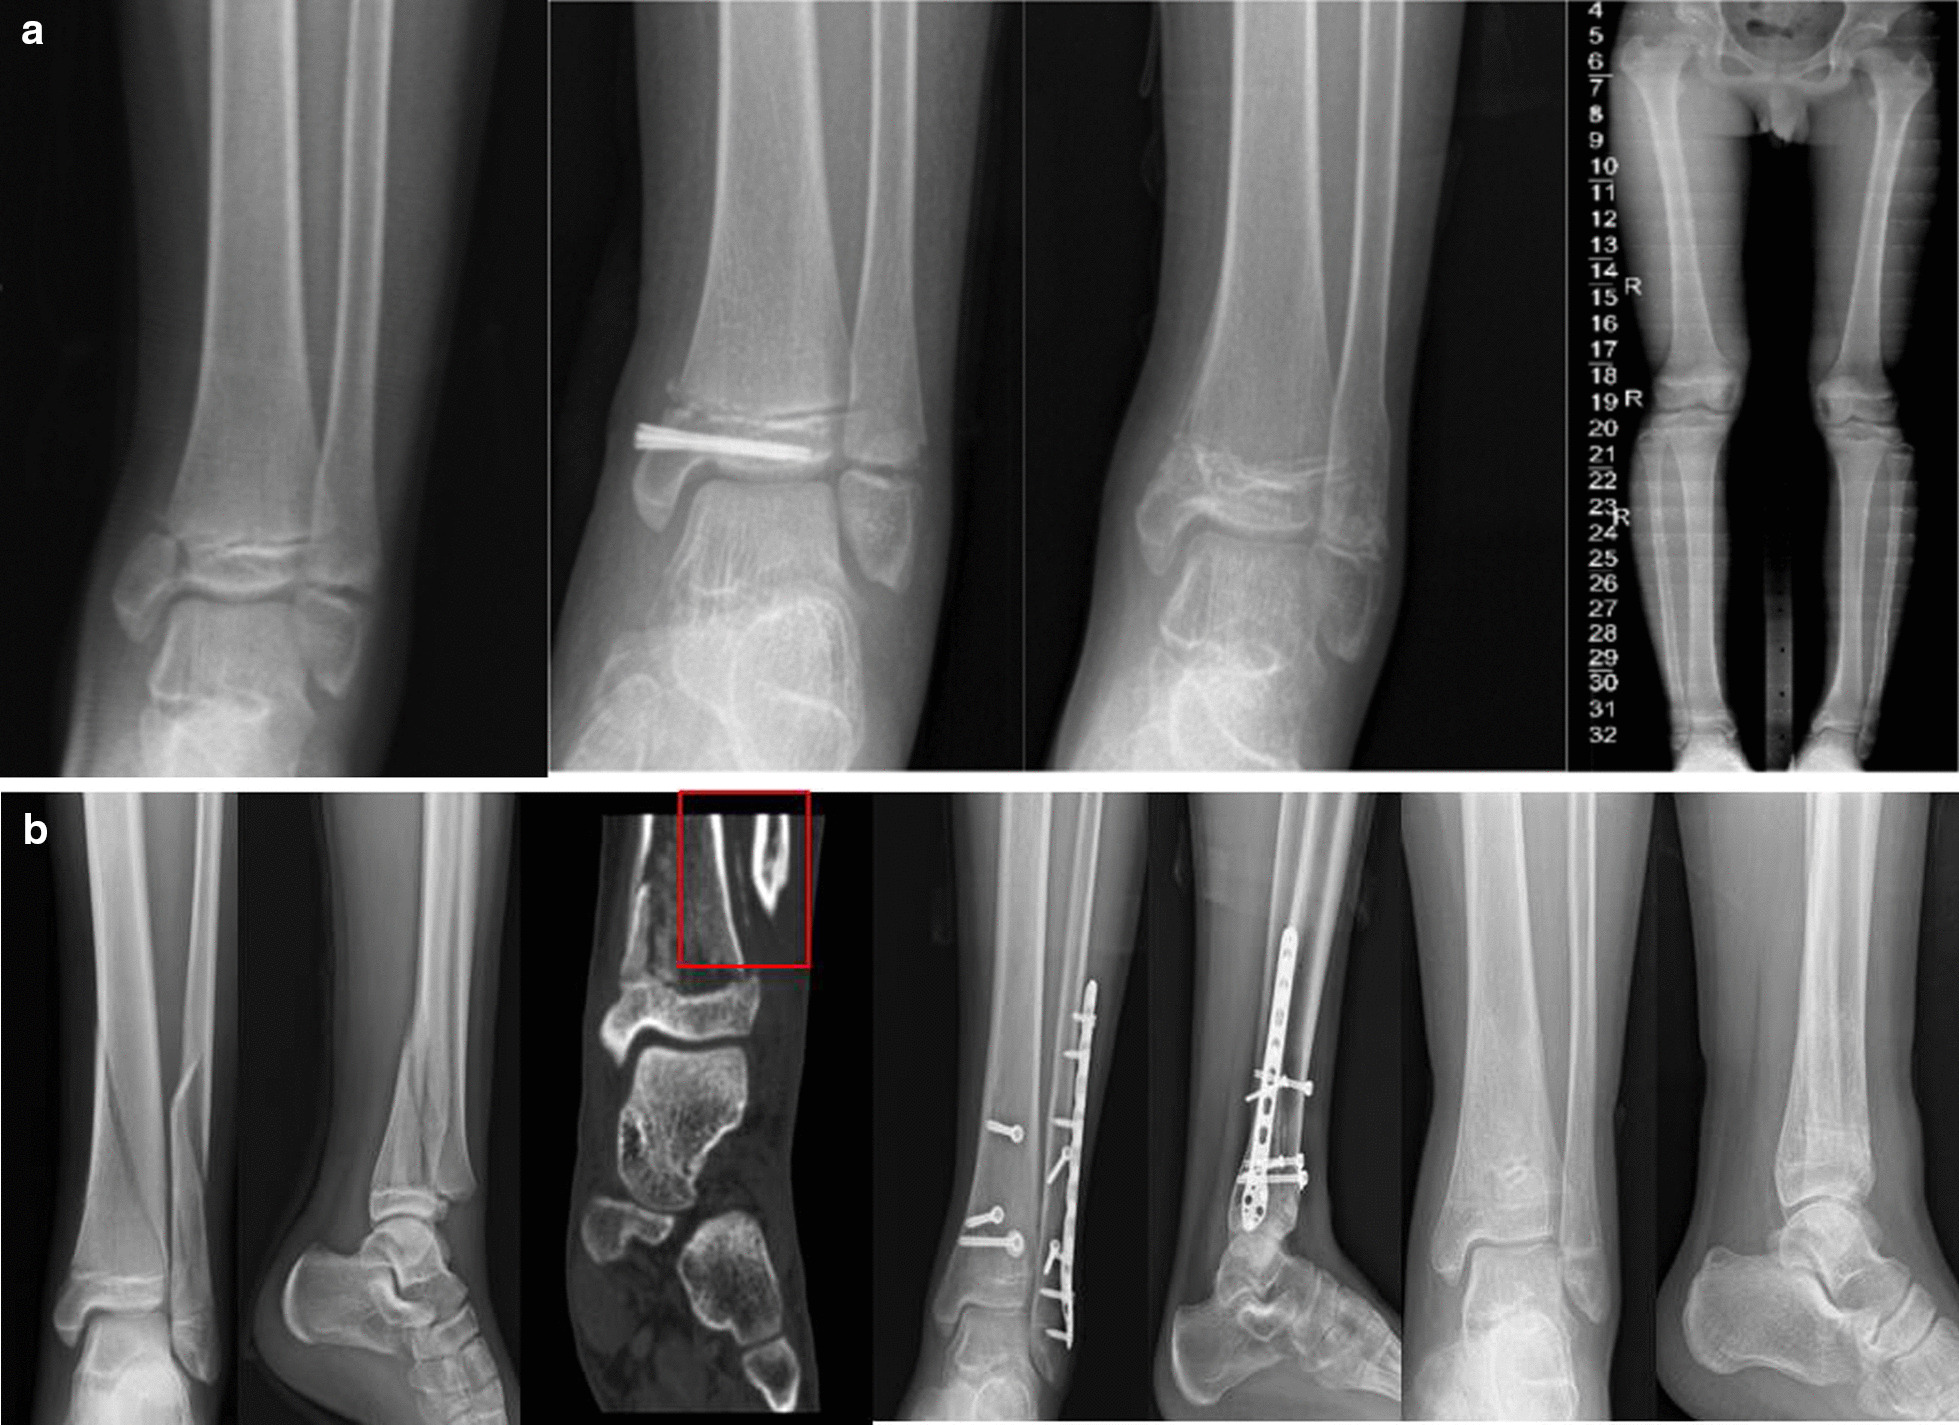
a An 11-year-old patient, Salter–Harris type II, distal tibia physeal fracture accompanied by fibula fracture, accepted open reduction 17 days after injury, osteotyluss growth can be seen in CT scan showed in the red frame. Growth disturbance has not been observed during 39 month follow-up. b An 11-year-old boy, Salter–Harris type III Distal tibia physeal fracture, accepted open reduction 4 days after injury. Growth disturbance has been observed at 13-month follow-up, the lateral angle of the distal tibia was 98° at 26-month follow-up
